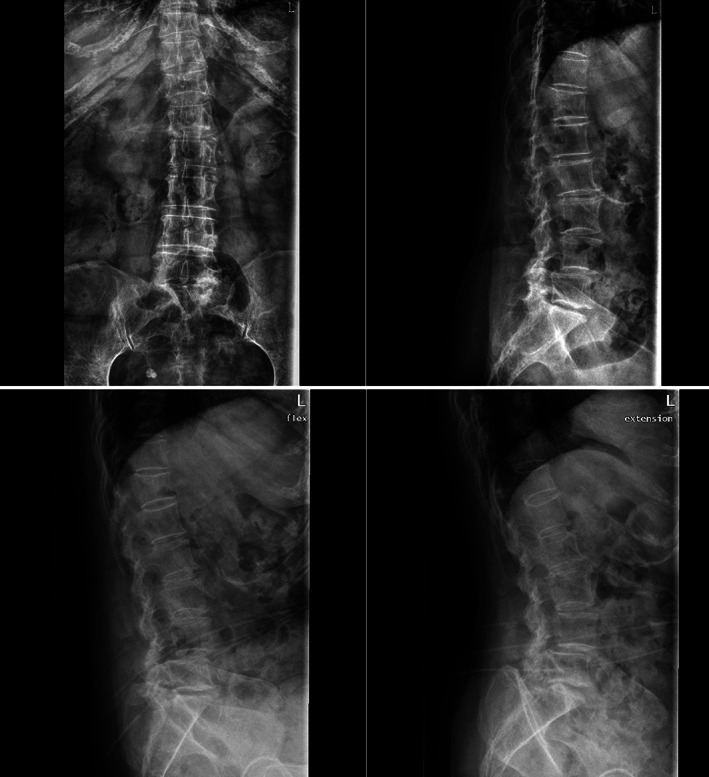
Preoperative static and dynamic anteroposterior radiograph of the lumbar spine. Mild forward slippage of L_4_, which manifested as instability in lumbar dynamic position, scoliosis, and degenerative change with osteoporosis.
